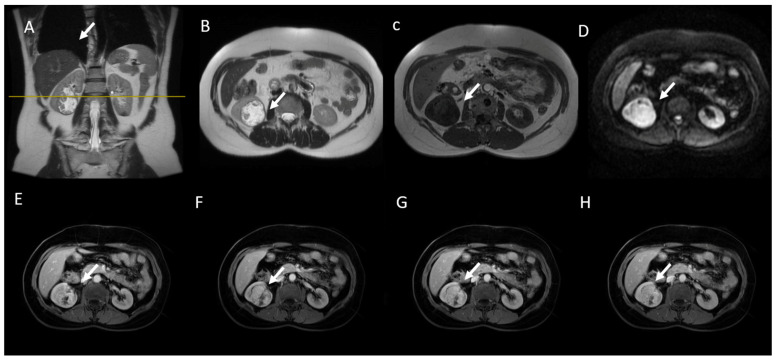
MRI assessment of RCC. (A) Coronal and (B) axial T2-weighted images show a heterogeneous hypointense renal mass (arrow). (C) Axial in-phase T1-weighted image shows a drop in signal intensity with restricted diffusion (D). Corticomedullary phase (E,F) and nephrographic phase (G,H) axial 3D fat-saturated GR T1-weighted images show mild enhancement.
